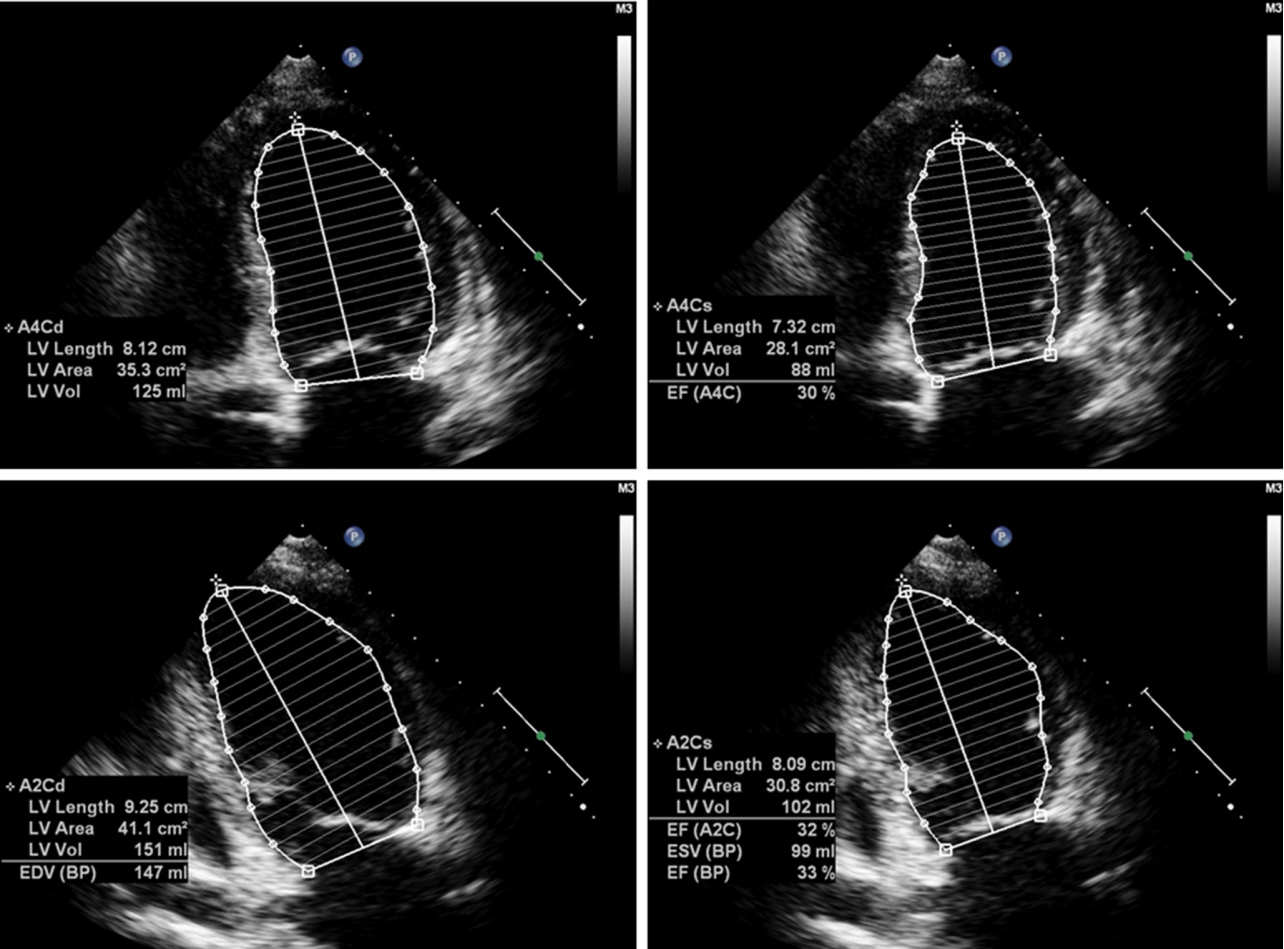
Restrictive cardiomyopathy on echocardiogram: note significantly decreased ejection fraction (EF) of 33 %, increased end-systolic volume (ESV) of 99 ml (normal <50 ml) and end-diastolic volume (EDV) of 147 ml (normal <120 ml)
